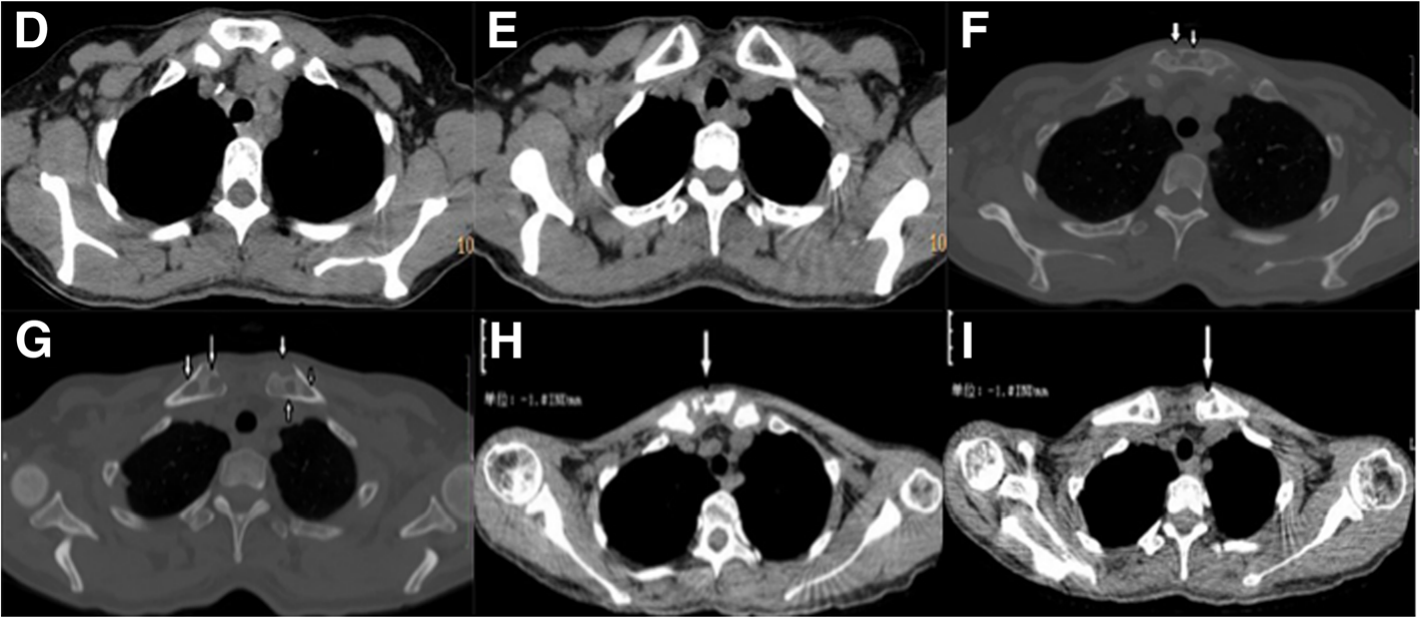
d-i Are chest computed tomography sequences. d and e were taken in August 2017, which showed no visible bone destruction in the sternum. f and g were taken in February 2018, which showed the bones of sternal stem and bilateral clavicle sternum stalk destructed. h and i were taken in June 2018, showing bone destruction of sternal stem and clavicle sternal stem
